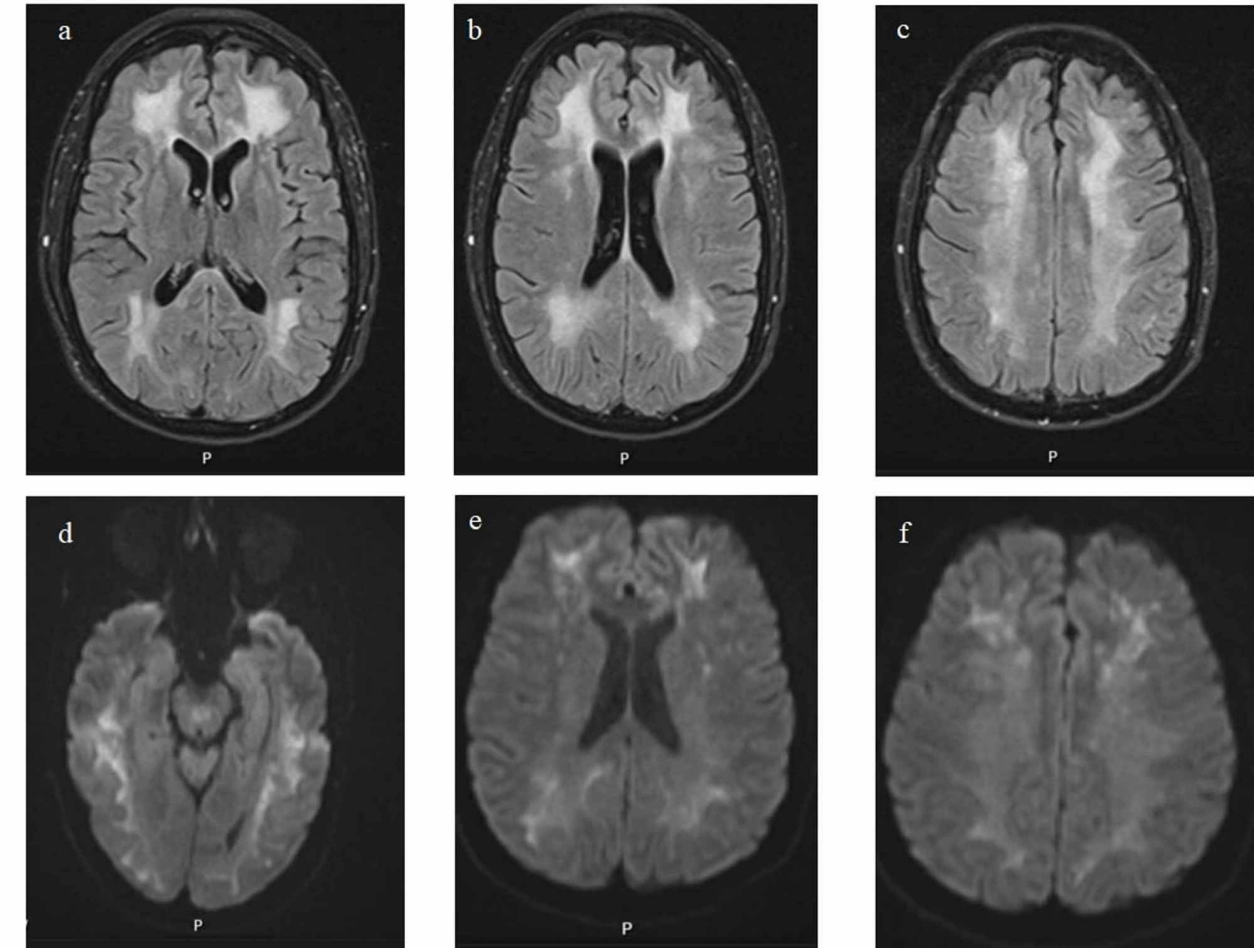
Brain MRI images demonstrate diffuse cortical and subcortical white matter diffusion restriction with corresponding FLAIR hyperintensities of the bilateral cerebral hemispheres A-C; DWI sequences. D-F: T2-FLAIR sequence. FLAIR - fluid-attenuated inversion recovery; DWI - diffusion-weighted imaging
